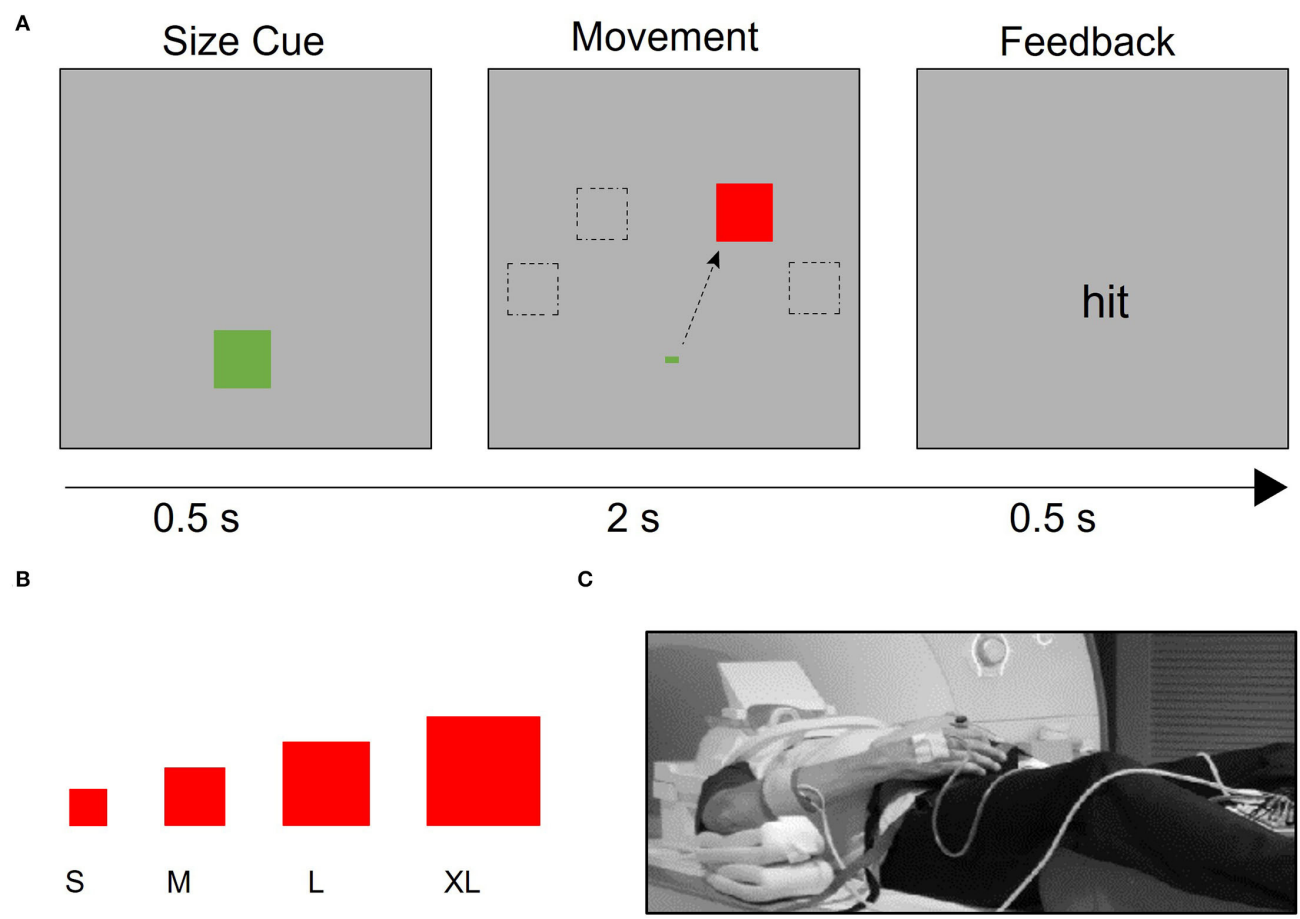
Task design. (A) After a target size cue, the target appears in one of four possible locations (−60°, −30°, 30°, and 60° relative to the vertical meridian). Participants were instructed to use the joystick to move the cursor to the target as quickly and accurately as possible. If the cursor began at the starting point and was inside the target 2 s after the target presentation, participants received “hit” feedback; otherwise, they received “miss” feedback. (B) Four different target sizes were used to vary the demand on precision, (C) Participants used an MRI-compatible joystick to perform the task. The joystick was secured to their body with straps and pads were placed to support the arm and minimize elbow and shoulder movement. EMG electrodes were placed on participants' right- and left- extensor carpi ulnaris (ECU) muscles, which support the manipulation of the joystick.
